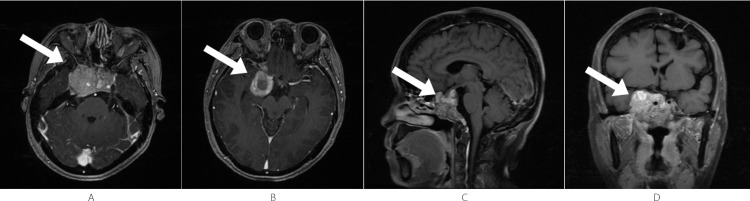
Sixteen Months Post-IMPT - March 2025 The sellar lesion is significantly smaller compared to the previous scan; the lesion anterior to the left midbrain is significantly smaller; compression of the brainstem and optic chiasm is significantly relieved. (A, B) Axial T1-weighted post-contrast images show that the sellar lesion is significantly smaller compared to previous scans; the lesion anterior to the left midbrain is significantly smaller (arrow). (C) Sagittal T1-weighted post-contrast image shows the lesion with heterogeneous mild-to-moderate enhancement (arrow). (D): Coronal T1-weighted post-contrast image shows the lesion with heterogeneous mild-to-moderate enhancement (arrow). IMPT, intensity-modulated proton therapy
